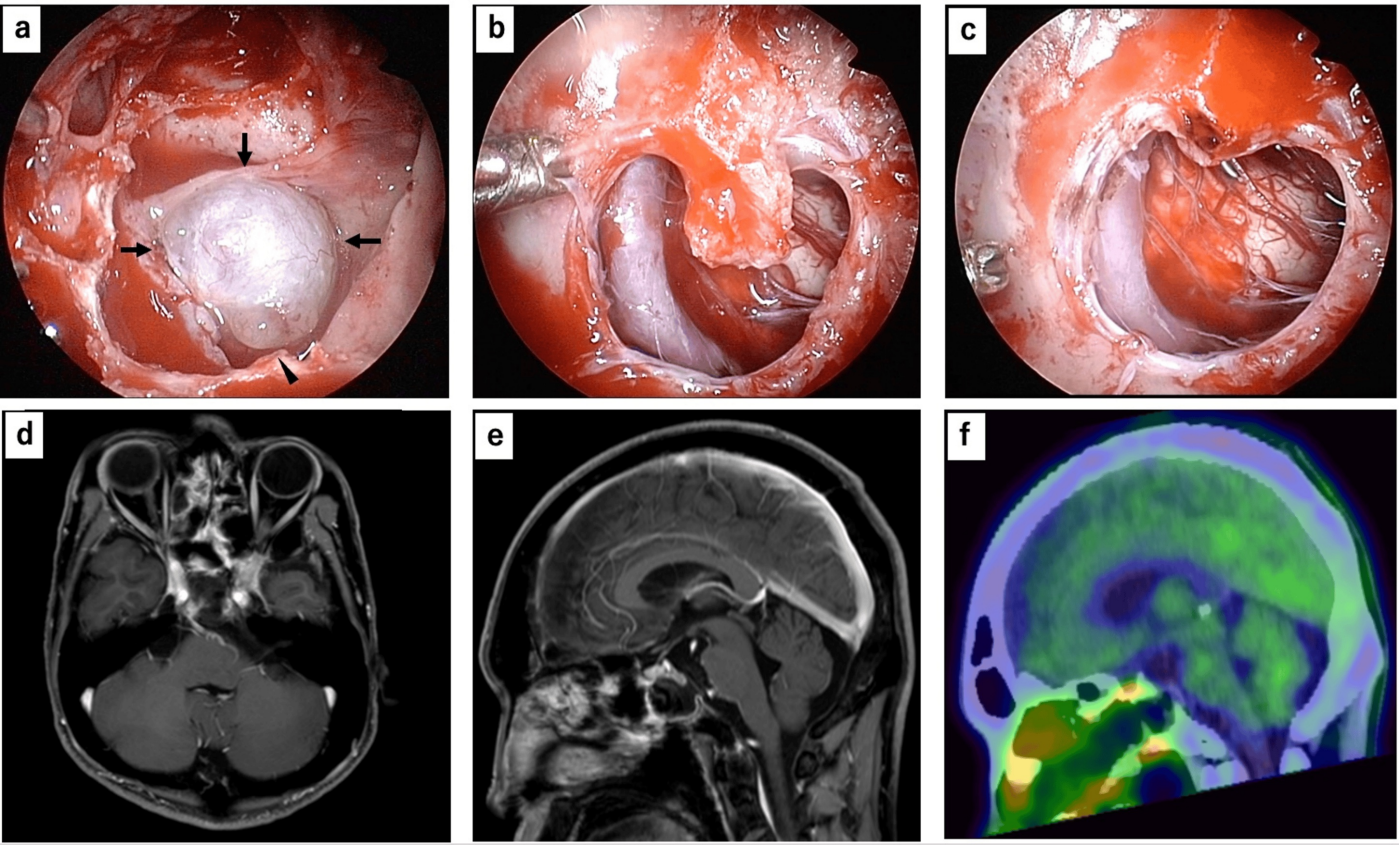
Intraoperative findings and postoperative imaging (a) The cyst wall protrudes into the sphenoid sinus through the clivus (black arrows). Cerebrospinal fluid leakage was observed from the inferior wall of the cyst (black arrowhead). (b) The cyst wall is removed and the prepontine cistern is observed through the defective clivus. (c) Gross total removal of the tumor is achieved and the thinned clivus is trimmed. (d, e) Postoperative magnetic resonance image showing no residual lesion. (f) 11C-methionine positron emission tomography computed tomography showing no methionine uptake around the clivus.
